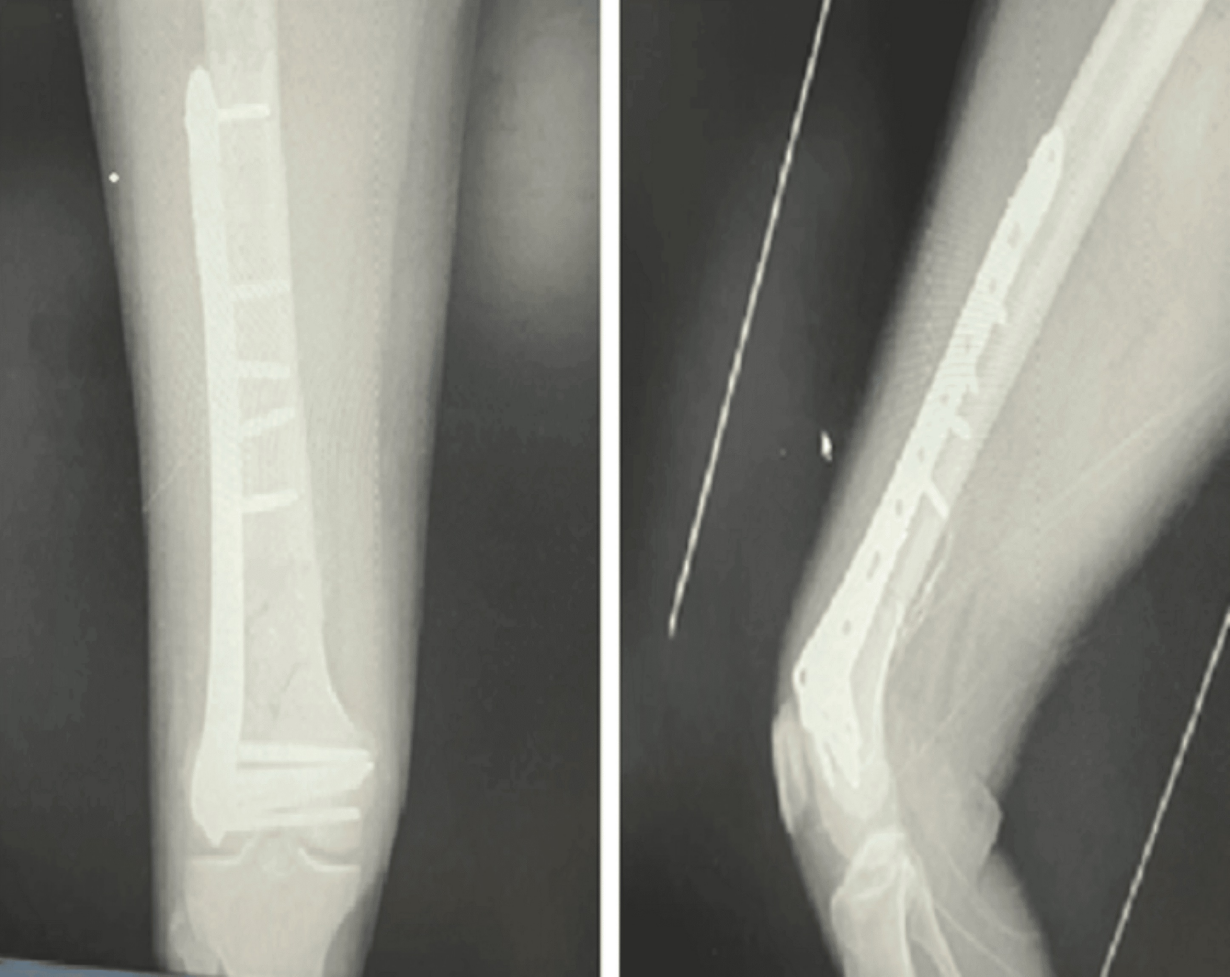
Case 1 X-ray at the six-month follow-up
